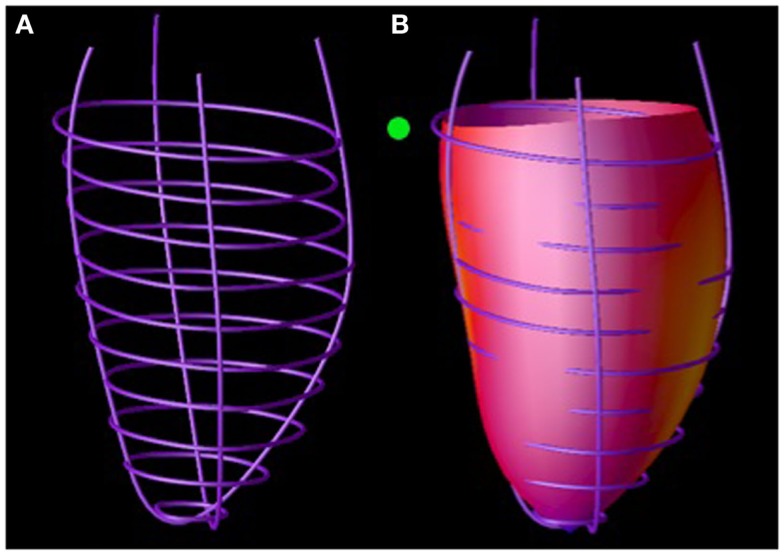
Septal wall curvature computation. LV endocardial contours manually traced on magnetic resonance images were positioned in 3D (A). A surface was fit to these contours (B). Septal wall curvature was computed from the surface curvature at the septal wall (denoted by the green ball).
